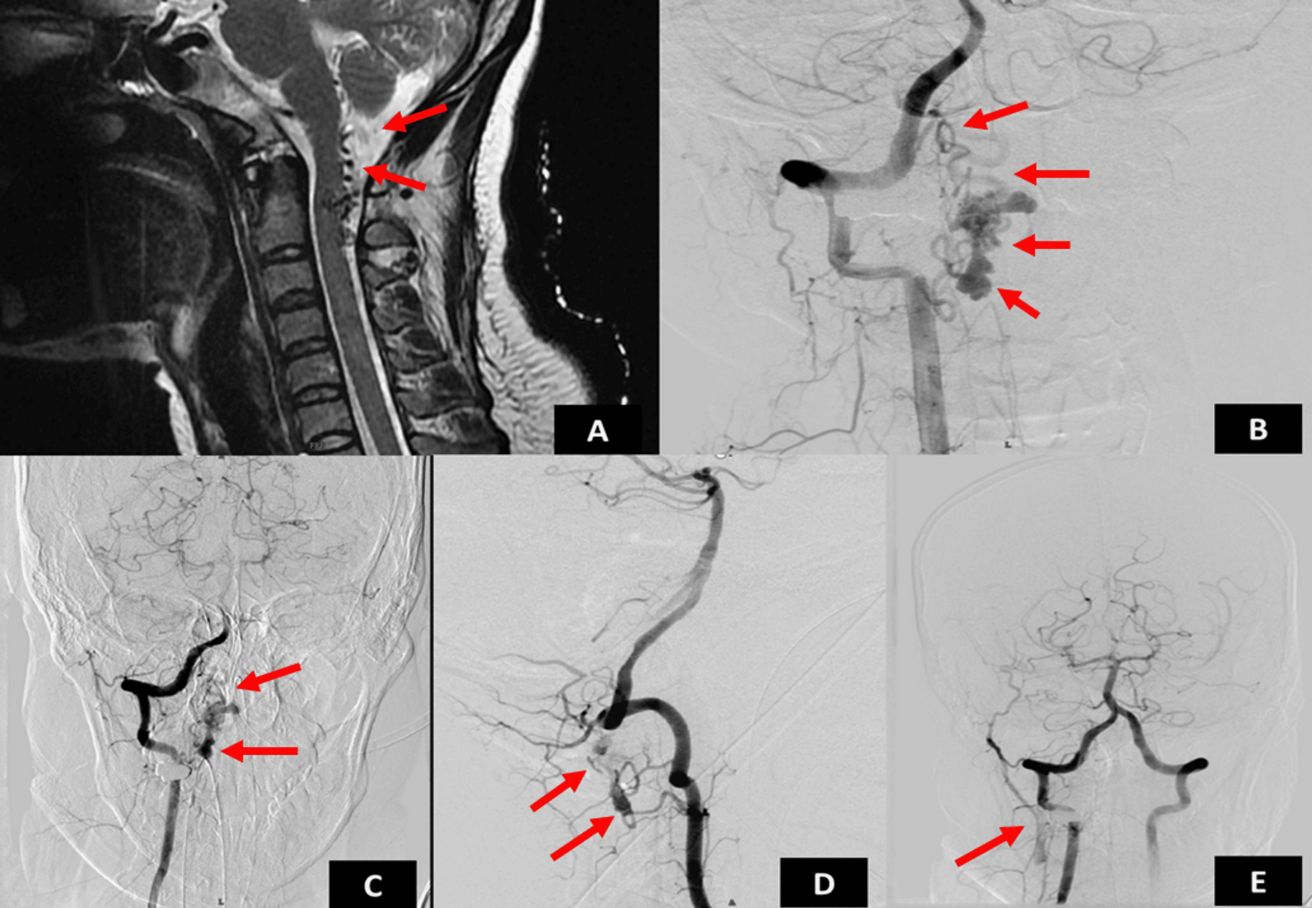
(A) Preoperative MRI of the patient. (B) DSA revealed AVMs in the intradural perimedullary (type IV- sub type II) C1-C2 segment of spinal cord. (C, D, E) Good occlusion of the AVM noted AVM: arteriovenous malformation; DSA: digital subtraction angiography; MRI: magnetic resonance imaging
